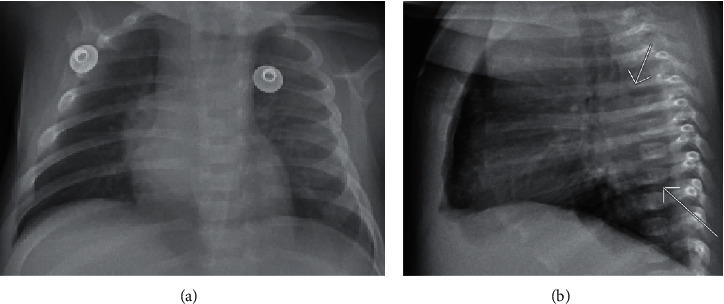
(a) Chest radiograph showing focal airspace opacity projecting over the right midlung zone. (b) Lateral CXR of “well-defined right lower lobe opacity interpreted as pneumonia versus mass.”
